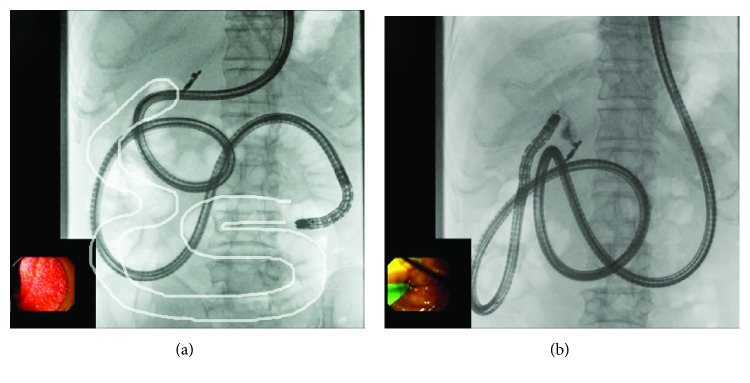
Roux-en-Y reconstruction without gastrectomy. A short-type SBE could not reach the blind end. (a) A short-type SBE could not reach the blind end because the length of the enteroscope was too short to adequately shorten the intestine. The white line indicates the course of the intestine up to the choledochojejunal anastomosis as confirmed during insufflation on radiography. (b) After switching to a long-type SBE, the intestine was successfully shortened. The blind end was reached.
